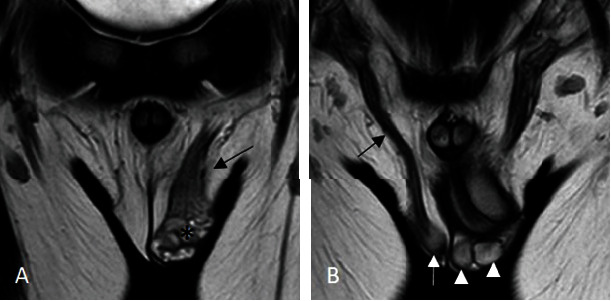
Magnetic resonance imaging in a seven-year-old boy diagnosed with polyorchidism. T2-weighted images in coronal sections, showing normally positioned right testicle (white arrow), and two testicles at the left side (white arrowheads) with homogenous and comparable hypersignal. Varicose scrotal veins are noted on the left side (star). A single epididymis and cord drained both left structures that was thicker (black arrow in (A)) and wider than on the right side (black arrow in (B)).
